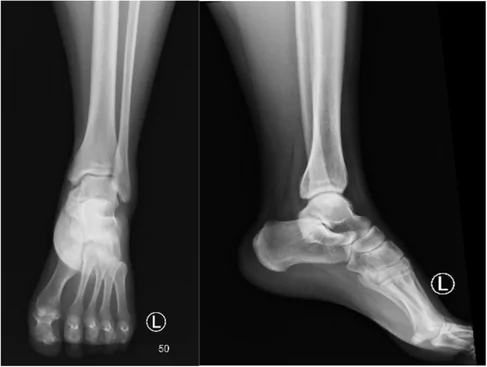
Preoperative plain radiographs of the left ankle in anteroposterior and lateral views showing no osseous abnormalities, fractures, or fibular groove deformities associated with peroneal tendon instability.
